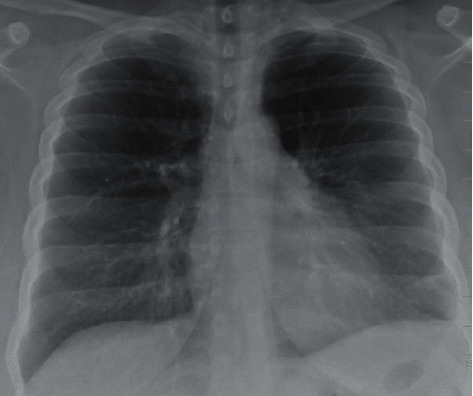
Chest X-ray revealing improved aeration of the lingula and left lower lobe with residual scarring and left pleural thickening.
